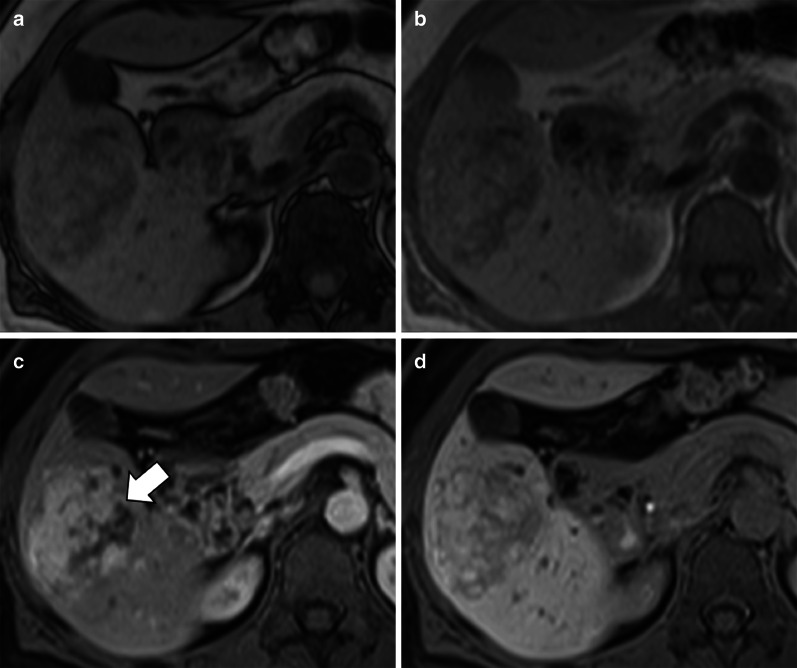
A 46-year-old woman with clinical history of oral contraceptive use and pathology-proven hepatocellular adenoma. Gadoxetate disodium-enhanced MRI shows a normal liver characterized by (a) no significant signal drop of hepatic parenchyma in the opposed phase compared to (b) the in-phase and (c) a hepatocellular adenoma (arrow) that shows contrast enhancement in the arterial phase and (d) heterogeneous hyperintensity in the hepatobiliary phase
